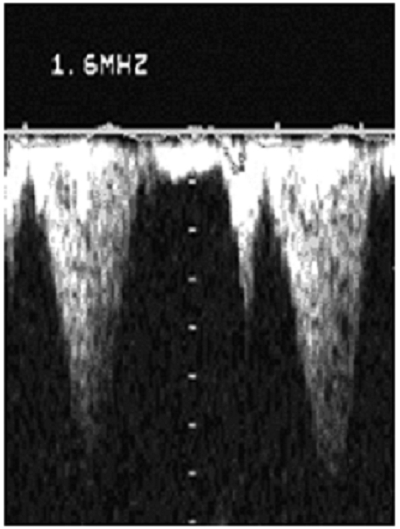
Cardiomyopathie hypertrophique obstructive; Gradient intra-ventriculaire en forme de lame de sabre en doppler continu
